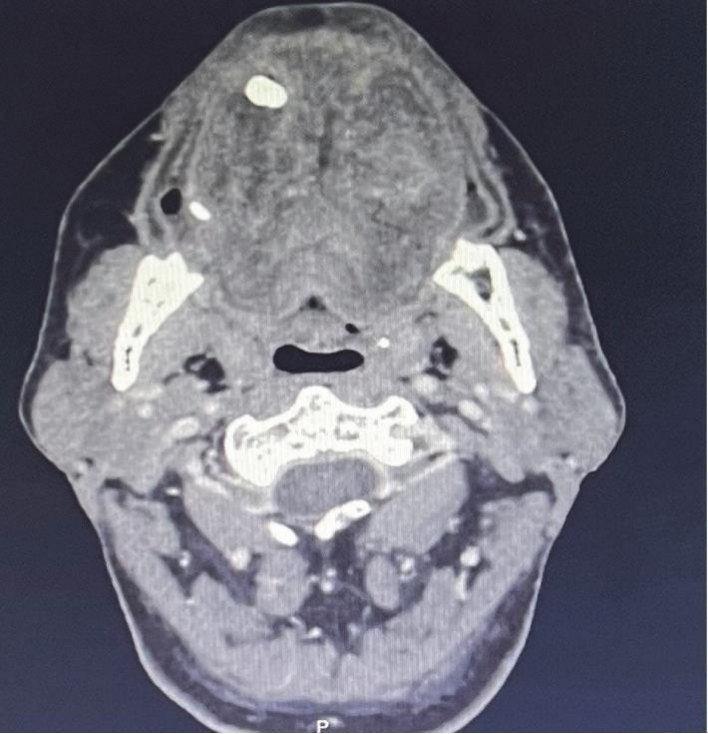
Axial CT scan of the oropharynx revealing heterogenous swelling of the tongue.
